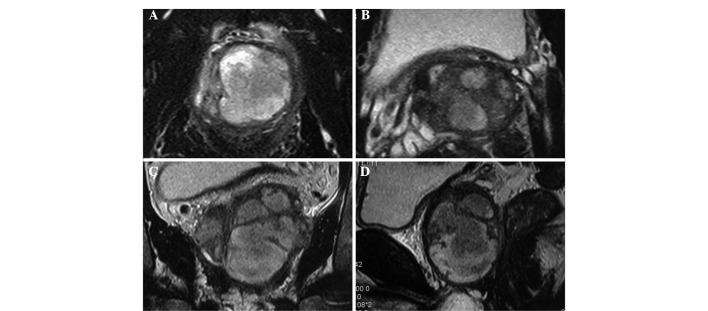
Magnetic resonance imaging exhibited an abnormal signal in the prostate and left seminal vesicle, indicating prostate abscesses involving the seminal vesicle. (A and B) Axial fat saturation images revealed multiple prostate abscesses involving the seminal vesicle; (C) coronal T2-weighted image; (D) sagittal T2-weighted image.
